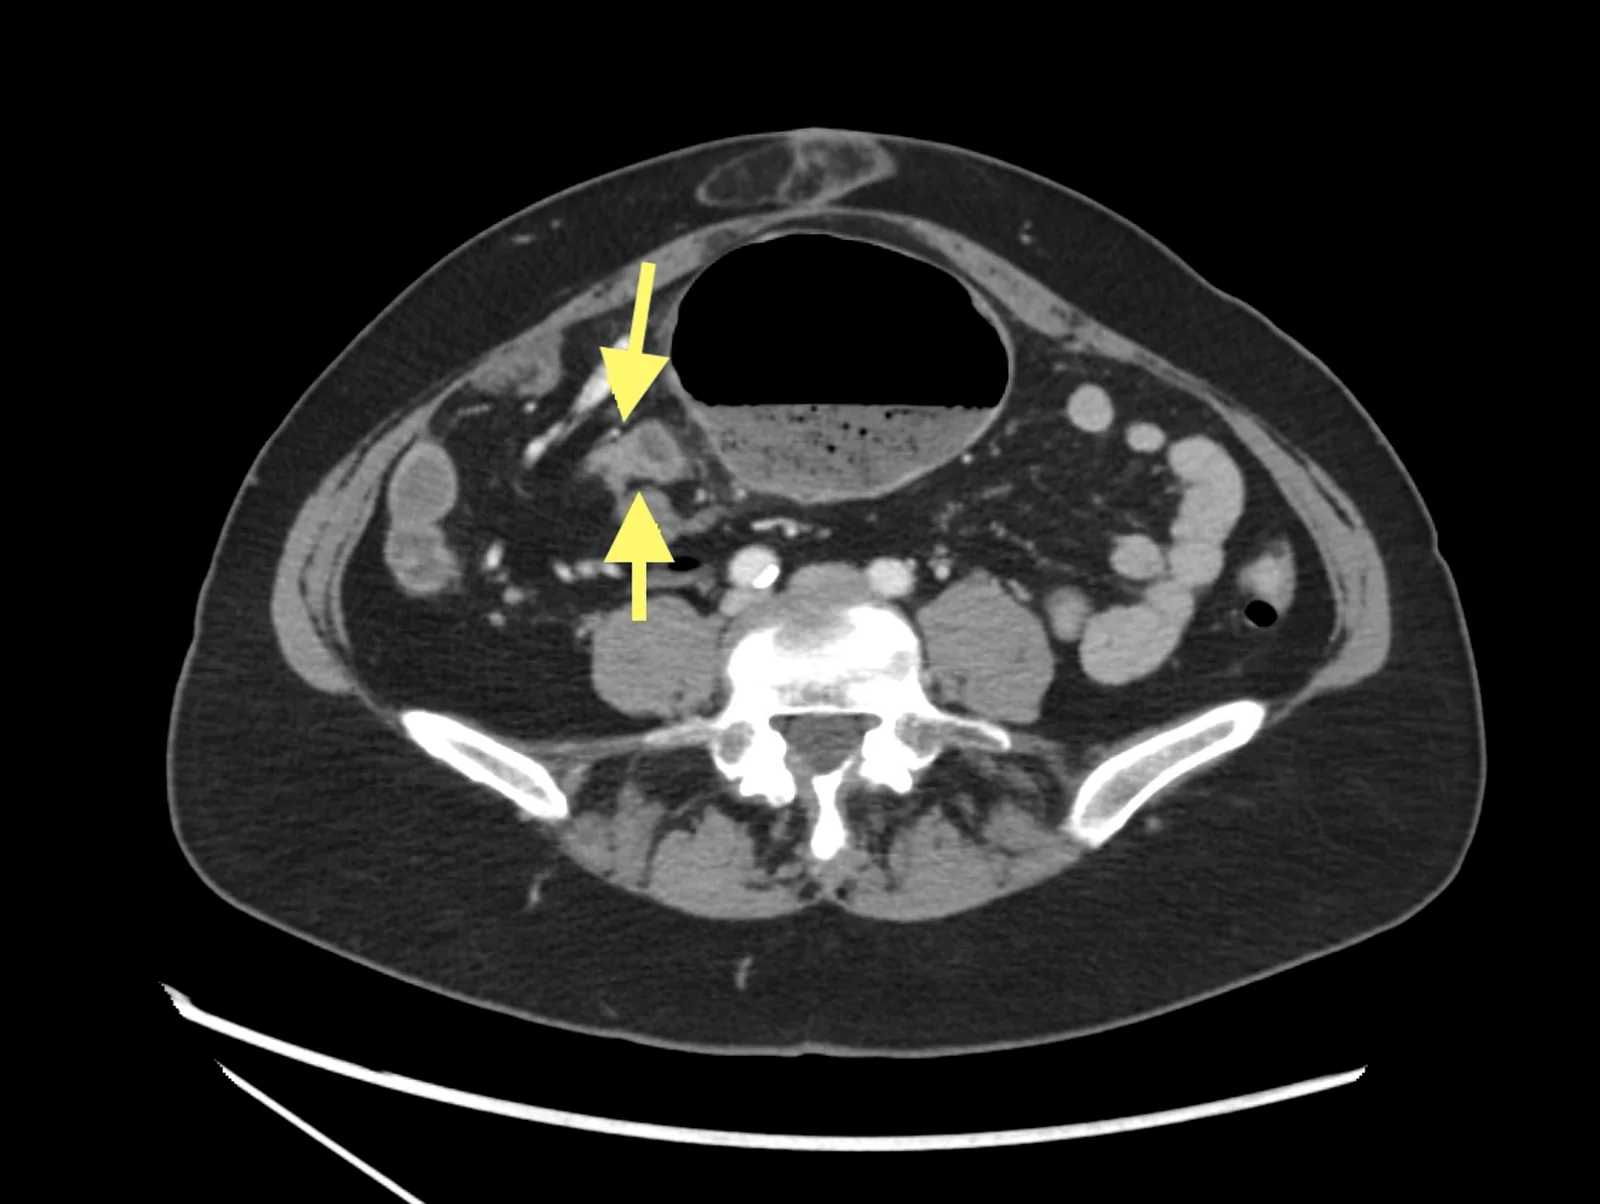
Axial CT image showing dilation of the cecum and a transition point raising concern for cecal volvulus. Axial contrast-enhanced CT image of the lower abdomen demonstrating a markedly distended, gas- and stool-filled cecum. The yellow arrows indicate mesenteric convergence at the transition point and adjacent fat stranding.
